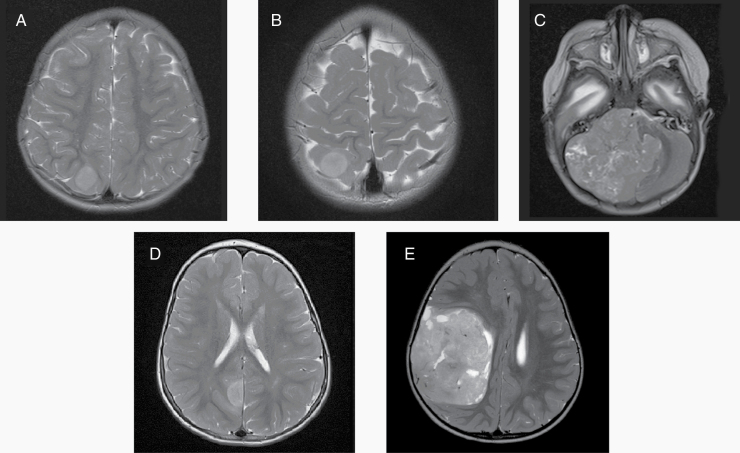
Representative MRI studies of patients. (A) A T2-weighted image of the brain from Case 1, demonstrating a hyperintense mass in the right parietal cortex. (B) A T2-weighted image of the brain from Case 2, demonstrating a lesion in the right superior parietal lobe. (C) A T2-weighted image of the brain from Case 3, demonstrating a large mass of the right posterior fossa. (D) A T2-weighted image of the brain from Case 4, demonstrating mass involving the right posterior parietal lobe. (E) A T2-weighted image of the brain from Case 5, demonstrating a large right fronto-parietal mass with midline shift.
